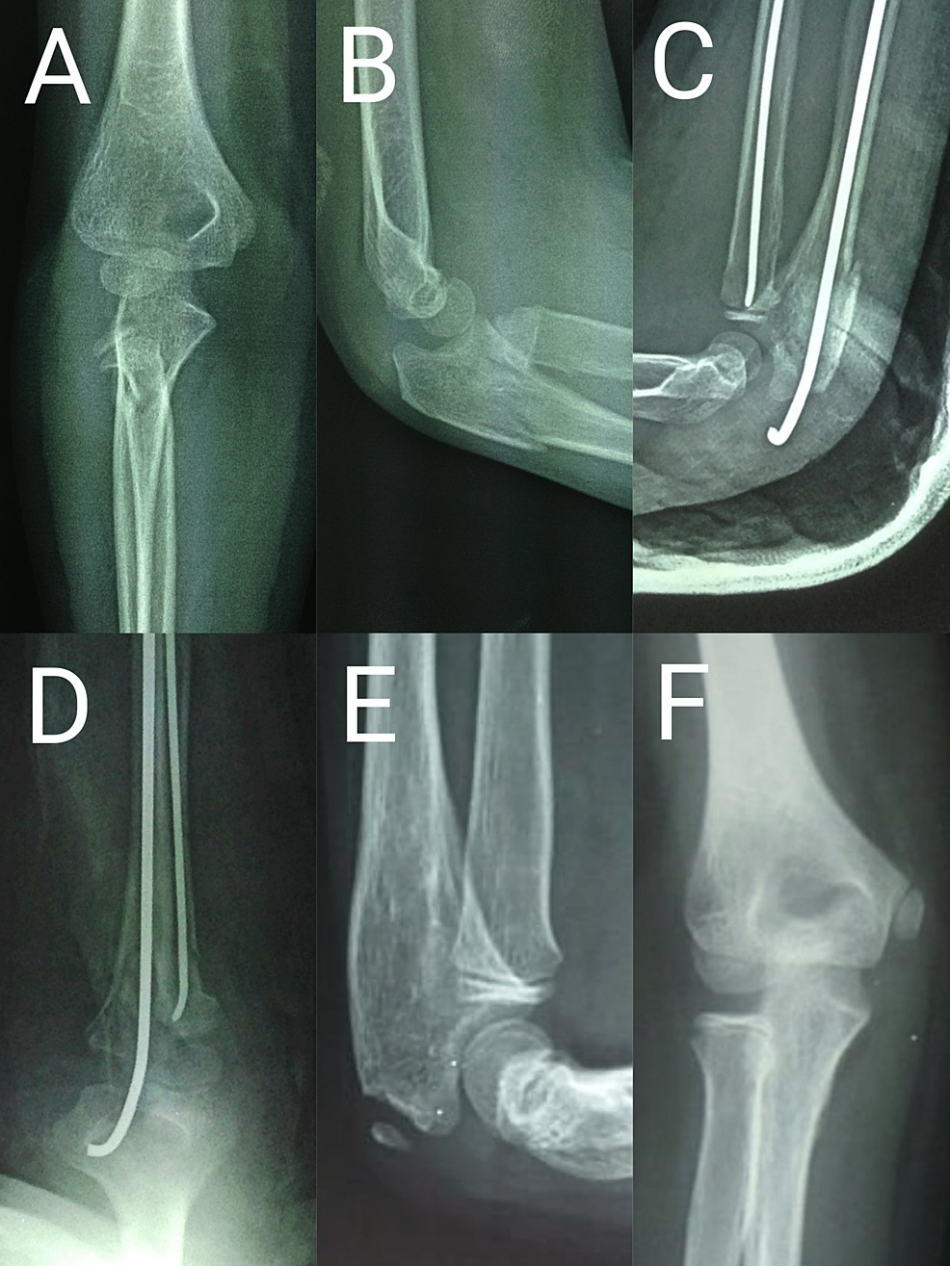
Radiographs of a nine-year-old male patient A and B: Preoperative radiograph of nine-year-old male showing Judet type III radial neck fracture and proximal ulna fracture; C and D: Immediate postoperative radiograph showing intramedullary nailing of radial neck fracture and K-wire fixation of ulna; E and F: Radiograph at the two-year follow-up showing fracture union
